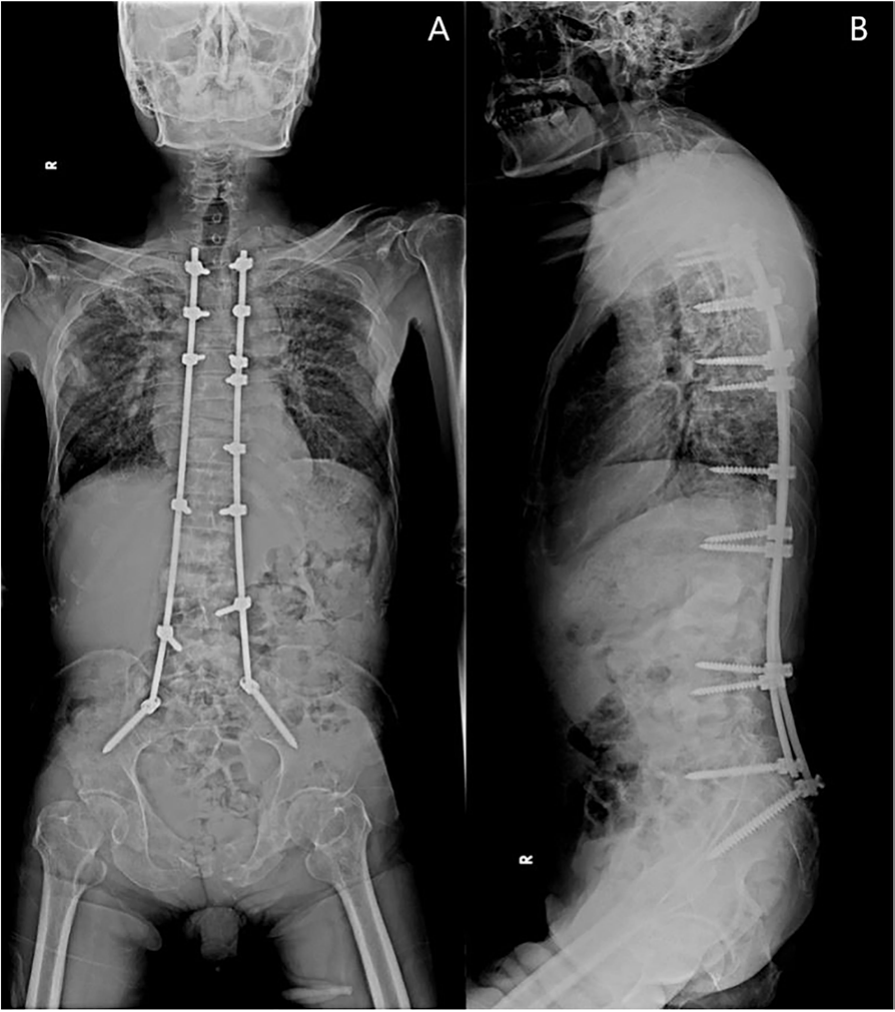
A Antero-posterior view of postoperative radiograph. B Lateral view of postoperative radiograph
